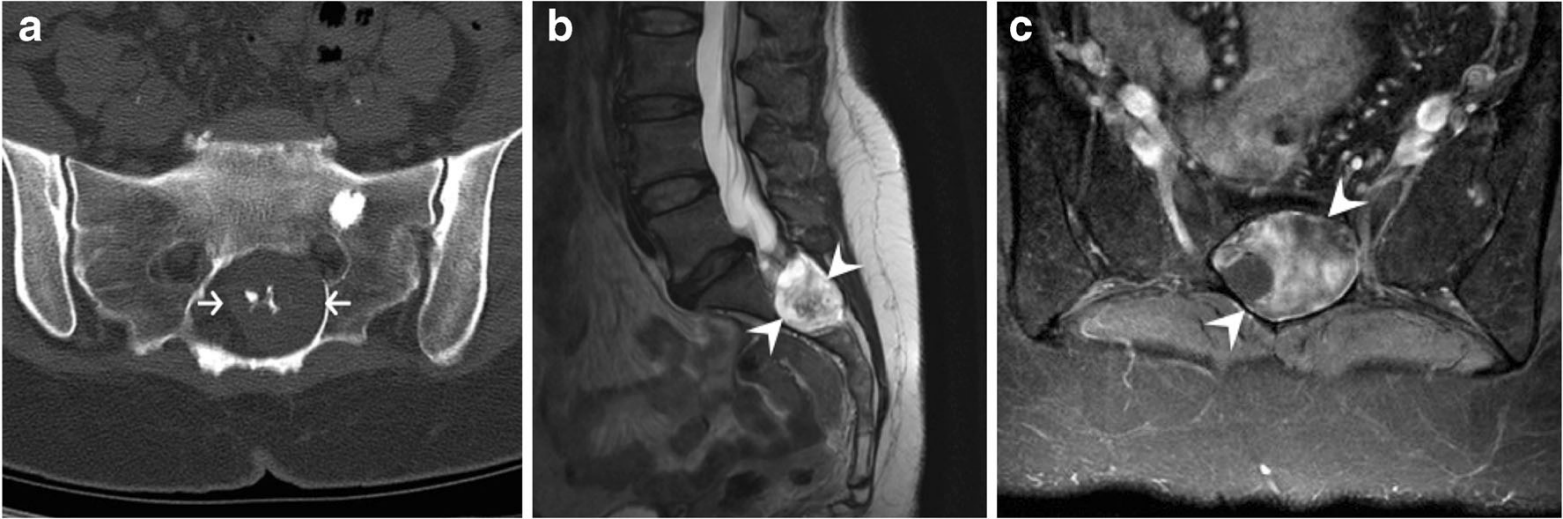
Schwannoma. (a) axial CT image of the sacrum shows calcifications in a lesion and (b) sagittal T2-weighted MRI image of the sacrum (arrows) and axial fat-saturated T1-weighted MRI image of the sacrum following gadolinium enhancement show the specific signal inside the schwannoma (arrowheads)
